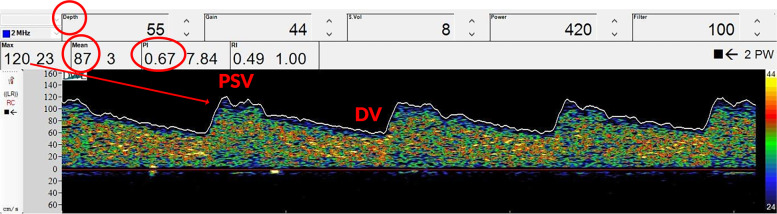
Representation of the transcranial Doppler ultrasonography showing cerebral blood flow velocity. PSV, peak systolic velocity; DV, diastolic velocity; MFV, mean flow velocity
